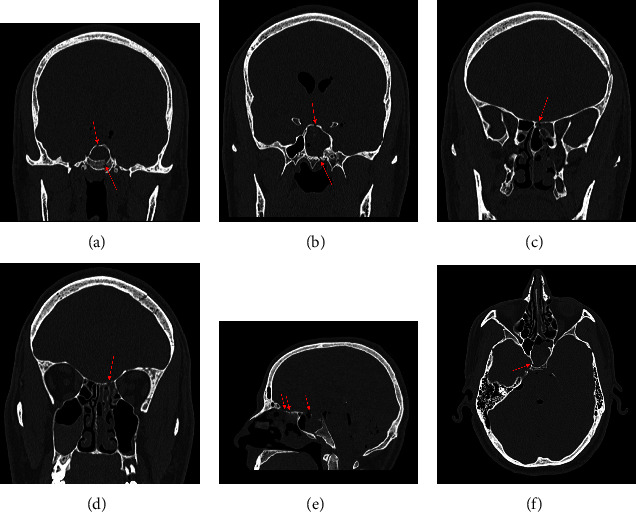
CT images of skull base defects. Red arrows point to skull base defects. (a) Preoperative coronal CT demonstrating defects in left sphenoid sinus and pneumocephalus. (b) Preoperative coronal CT demonstrating defects in left sphenoid sinus. (c) Preoperative coronal CT demonstrating defects in posterior left ethmoid roof. (d) Preoperative coronal CT demonstrating defects in posterior left anterior ethmoid roof. (e) Preoperative sagittal CT demonstrating multiple defects in the roof of the left ethmoid and sphenoid sinuses. (f) Axial CT demonstrating fracture of posterior wall of sphenoid sinus.
